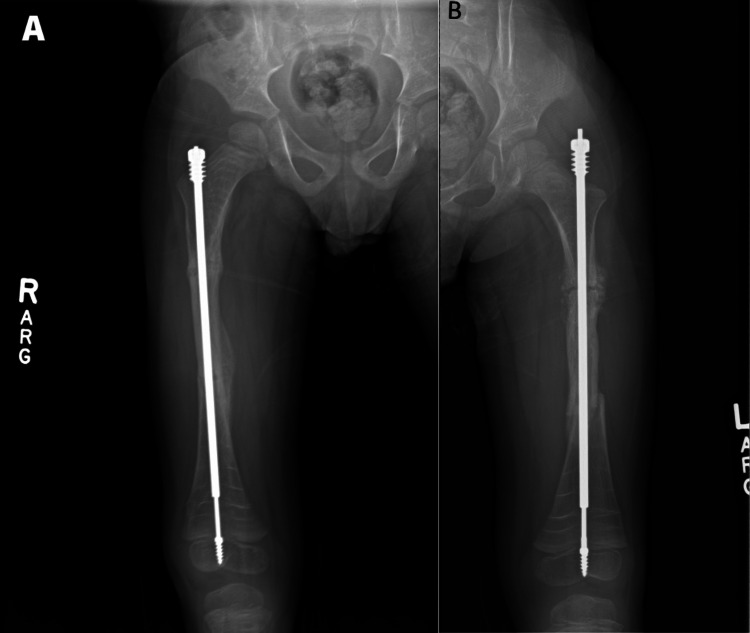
Postoperative radiographs demonstrating proper alignment of the implants and appropriate healing eight weeks after the operation.
